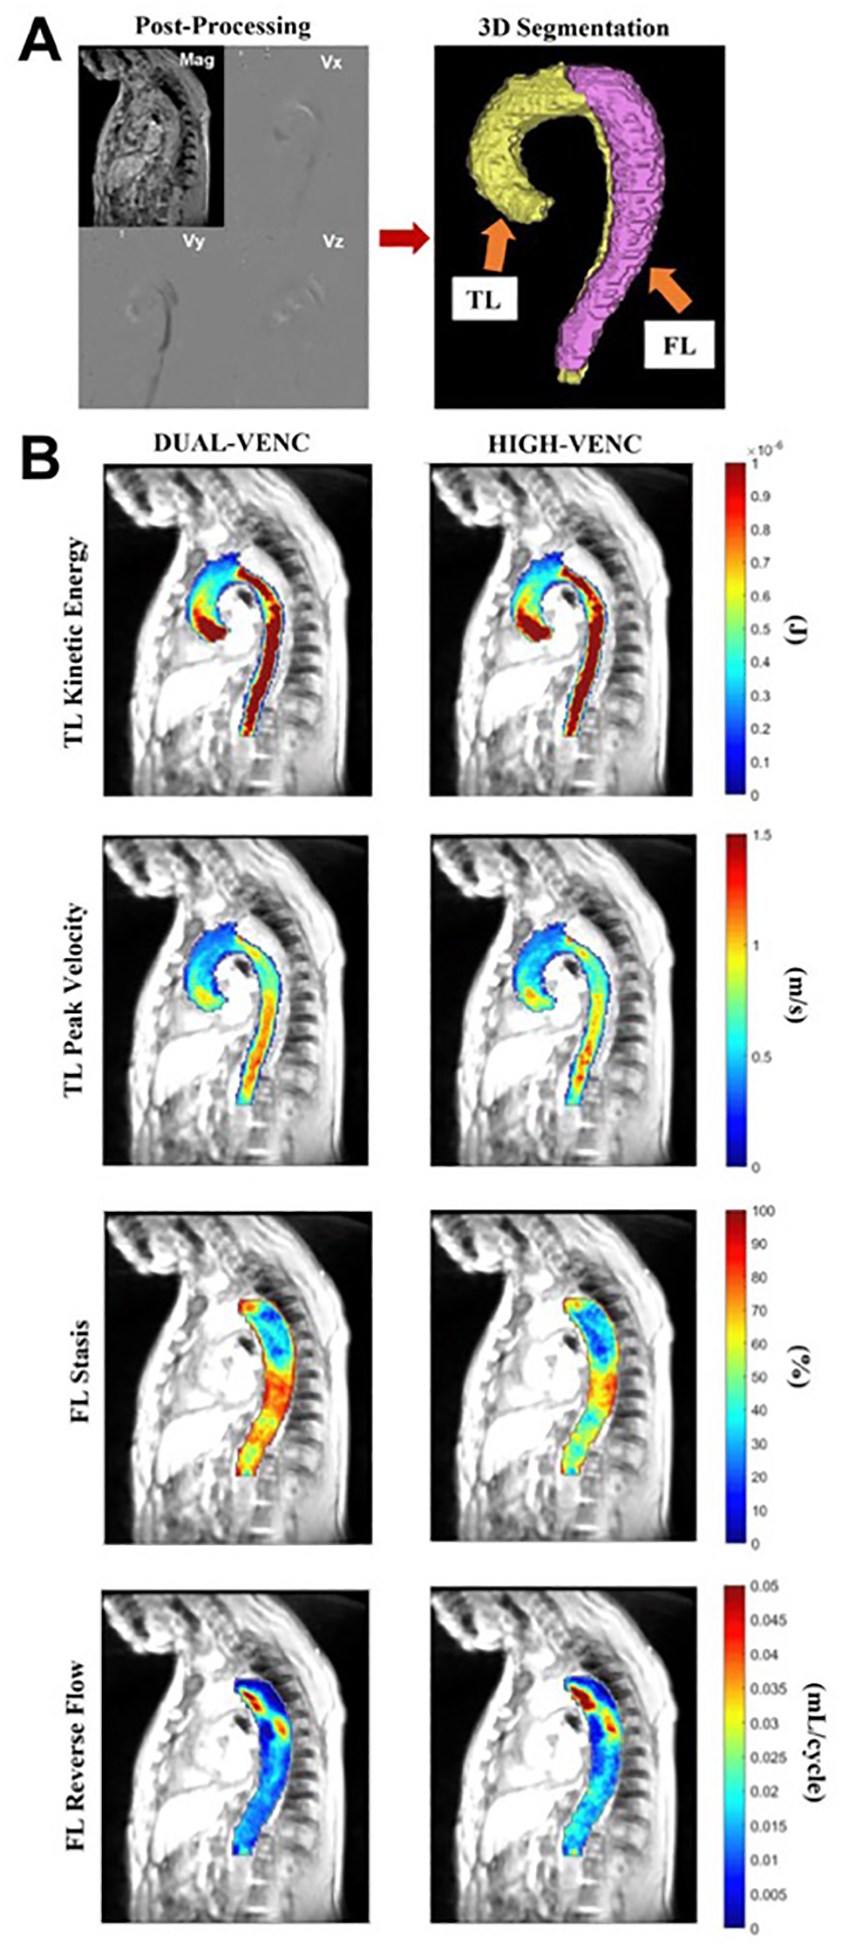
(A) Postprocessing of the 4D flow magnetic resonance imaging (MRI) data on MATLAB and manual true (TL) and false lumen (FL) 3D segmentations. (B) The volumetric maps of peak velocity and kinetic energy in TL and reverse flow and stasis in FL from the dual-venc (DV) acquisition and high-venc data extracted from the DV acquisition in one subject with de novo type B aortic dissection.
